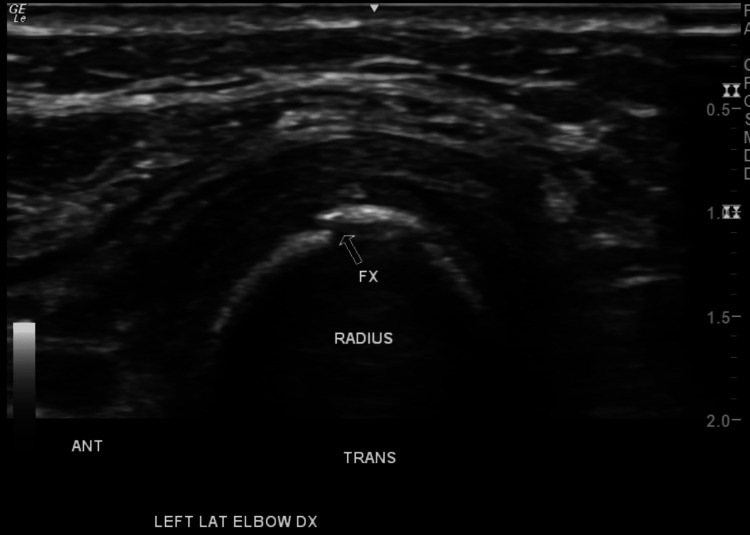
Forearm ultrasound with arrow showing the location of the fracture in a transverse view Ultrasound image of the patient’s left forearm in a transverse view. The figure shows a 1.5 mm cortical offset, indicating a possible fracture along the radial shaft distal to the radial head from a transverse view.
